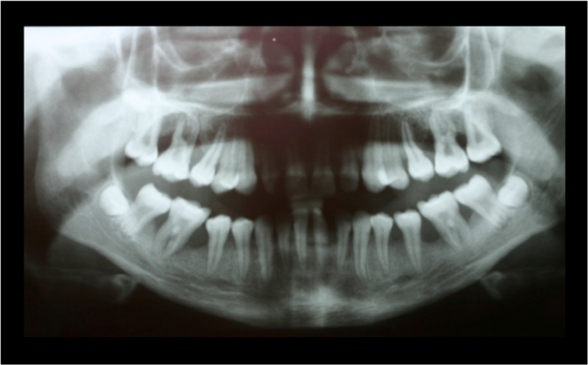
Panoramic radiograph at the age of 13 years: no periodontal therapy was rendered since the age of 9.
